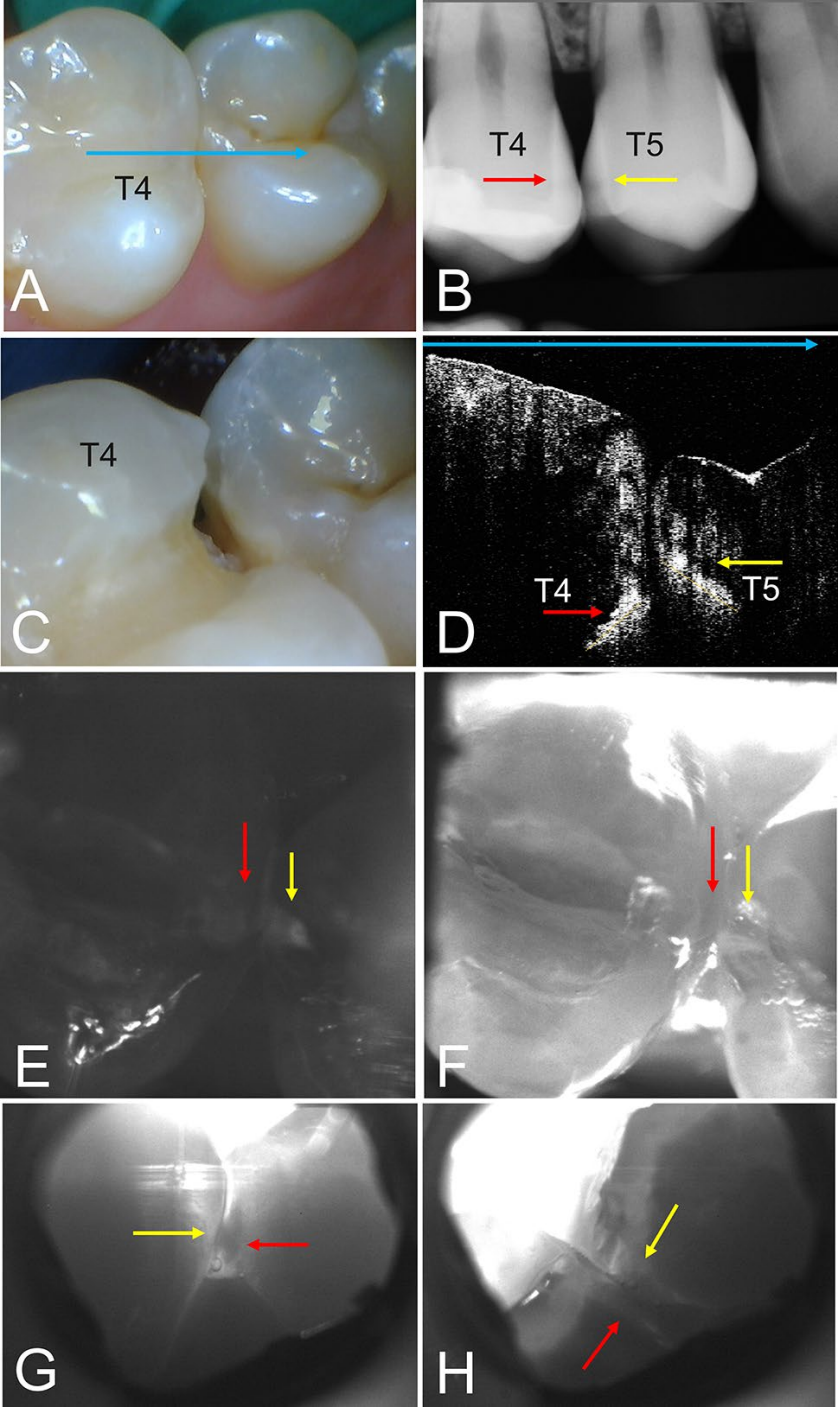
(A) Color image of the contact between tooth #4 (T4) and tooth #5 (T5), no lesions are visible. (B) Radiograph shows a lesion (red arrow) on tooth#4 at the distal contact that was scheduled for restoration and on the opposite contact on tooth#5 (yellow arrow). (C) After the cavity preparation decalcification is visible on the mesial contact of tooth#5. (D) A CP-OCT b-scan image taken from the occlusal surface above the contact along the path of the blue arrow shown in (A) shows both proximal lesions that appear with increased subsurface reflectivity at the positions indicated by the red and yellow arrows. The CP-OCT scan shows the intensity with depth and lateral position with white high intensity and black low intensity. (E) SWIR-R and (F) SWIR-OT images show lesions on both surfaces (red and yellow arrows). SWIR-PT images taken from the (G) buccal and (H) lingual sides show both proximal lesions across the contact
